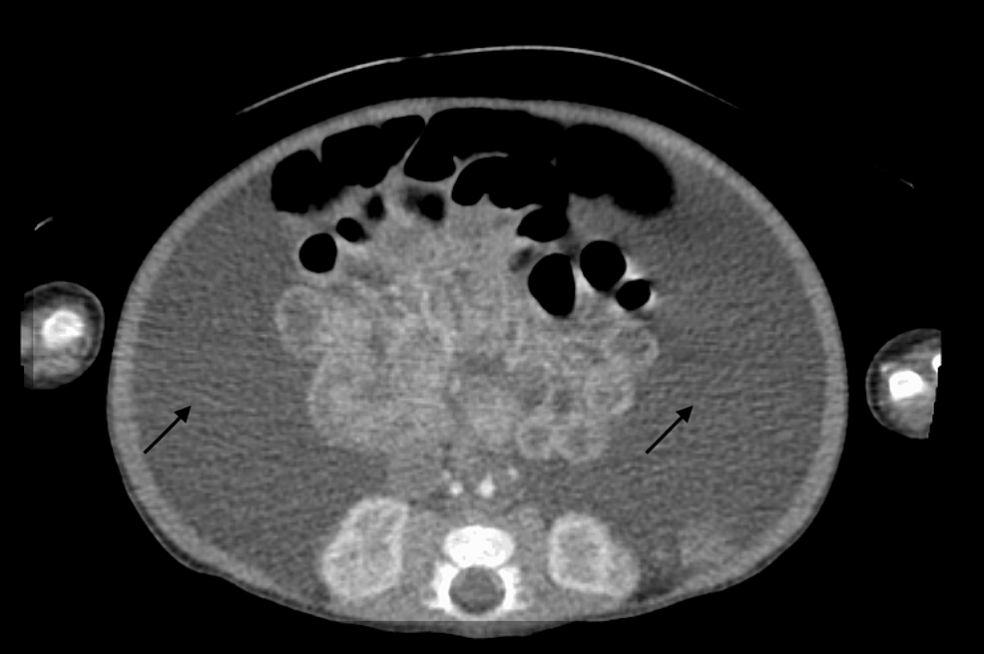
Large volume of chylous ascites on abdominopelvic computed tomography scan. Arrows indicate areas with chylous ascites.
